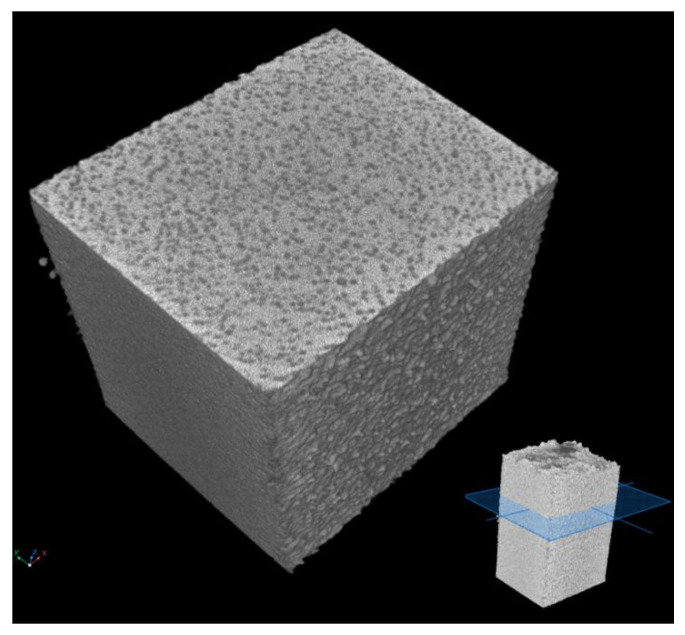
CT image of a ductile iron sample.
